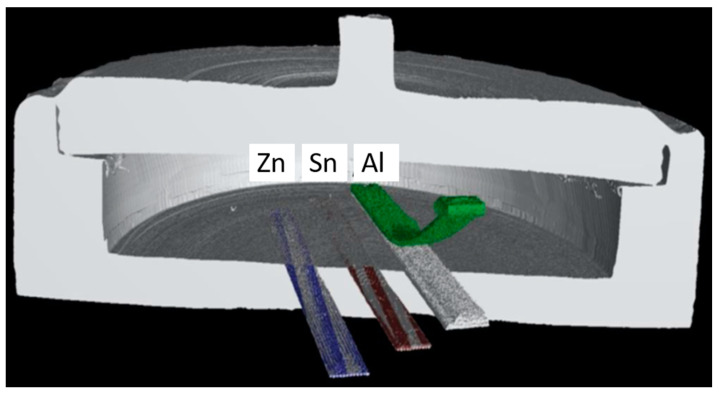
Cross section of a post furnace X-ray micro-computed tomography (XCT) image with zinc (blue), tin (maroon), superimposed over pre-furnaced image of a container sealed in air. Melt wires without color were taken before the furnace test. Melt wires with color were taken after exceeding the melting point of all three materials indicating no visible changes on two out of three wires when encapsulated in air.
